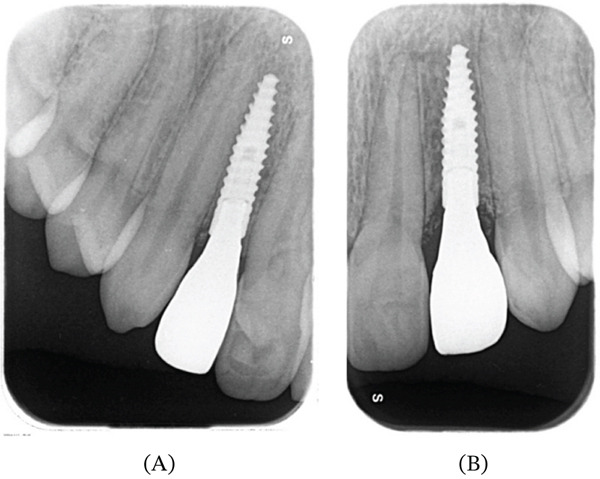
(A, B) Periapical radiographs.
